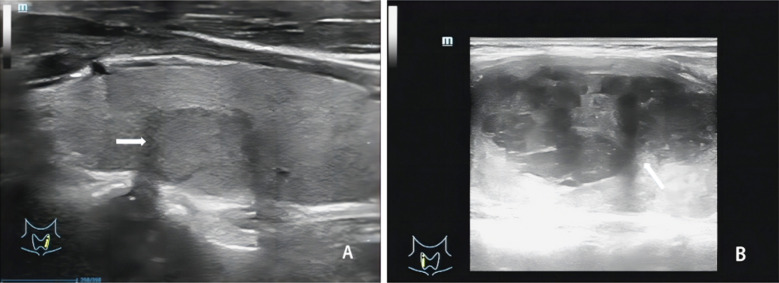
Ultrasound examination of benign and malignant ovarian tumors. A: The imaging features of benign group patients on ultrasound examination are mainly multiple masses with a diameter of<3cm and accompanied by high echogenicity; B: The imaging features of malignant group patients on ultrasound examination are mainly solitary masses with a diameter of ≥ 3cm and accompanied by hypoechogenicity.
